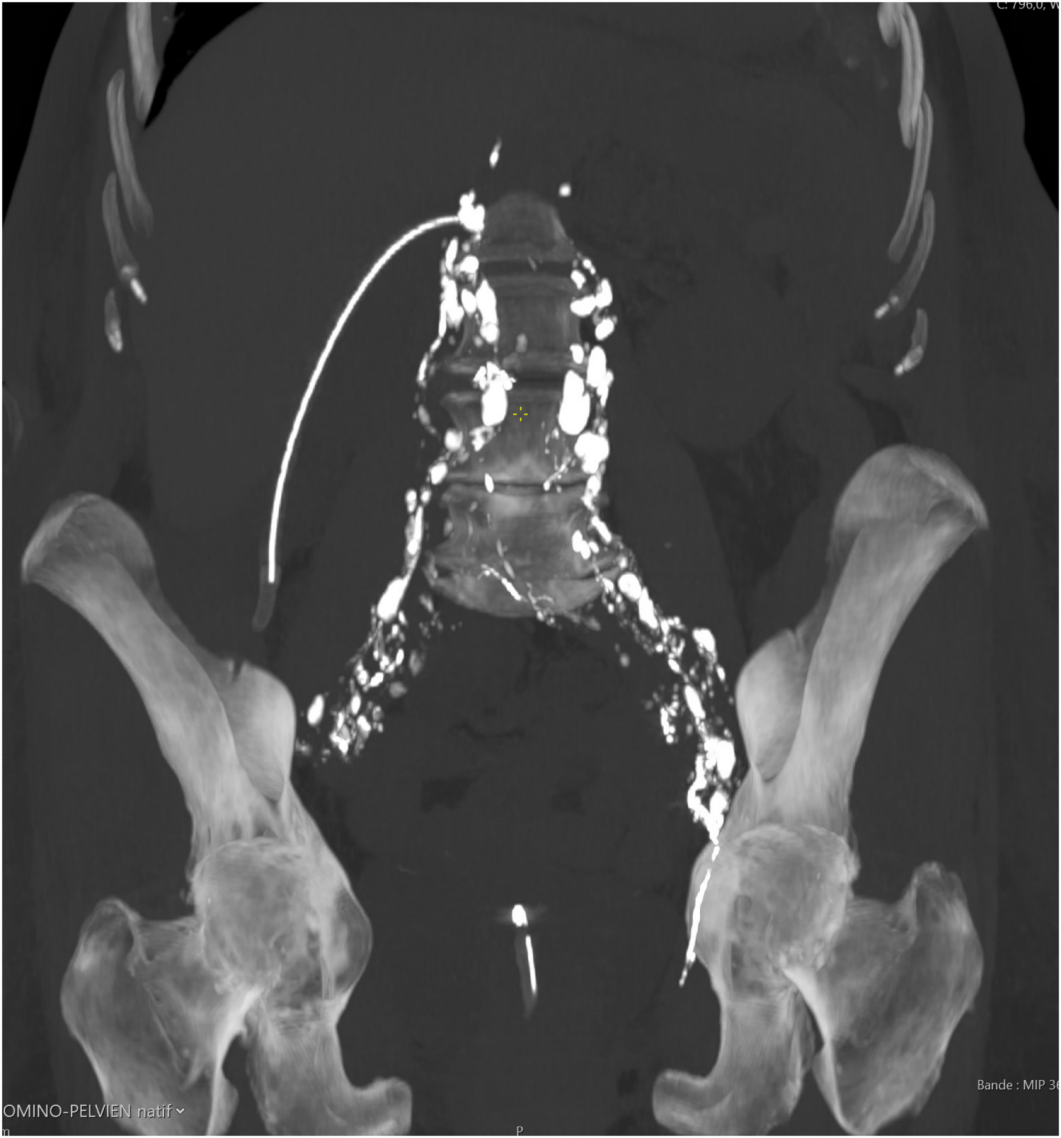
Axial CT scan after intranodal Lipiodol® demonstrating dense opacification of pelvic and paraaortic lymphatics without evidence of leakage.
